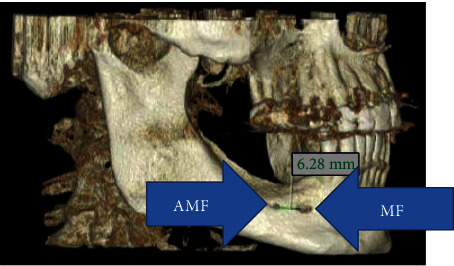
Right AMF at reconstructed CBCT.
